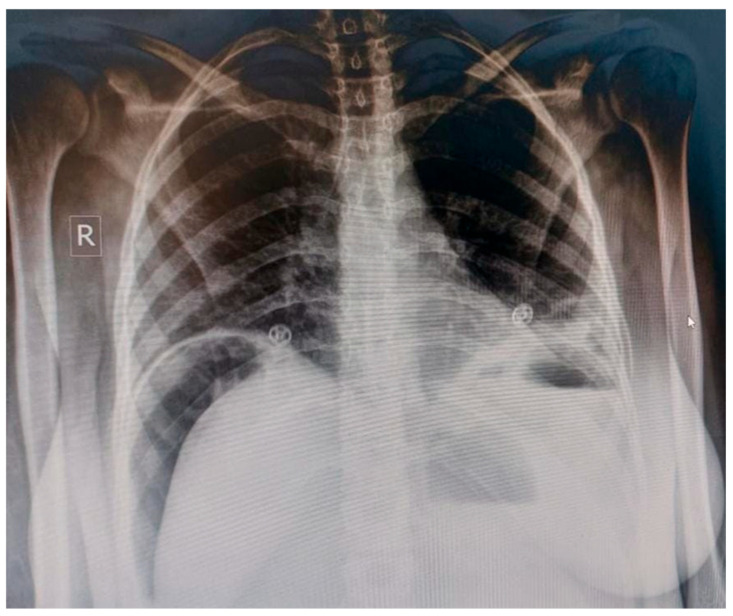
Abdominal radiograph demonstrating pneumoperitoneum. Erect abdominal X-ray showing free subdiaphragmatic air (arrow) consistent with perforated viscus, alongside dilated bowel loops and diffuse subcutaneous edema. These findings raised suspicion for intra-abdominal perforation prior to exploratory laparotomy.
